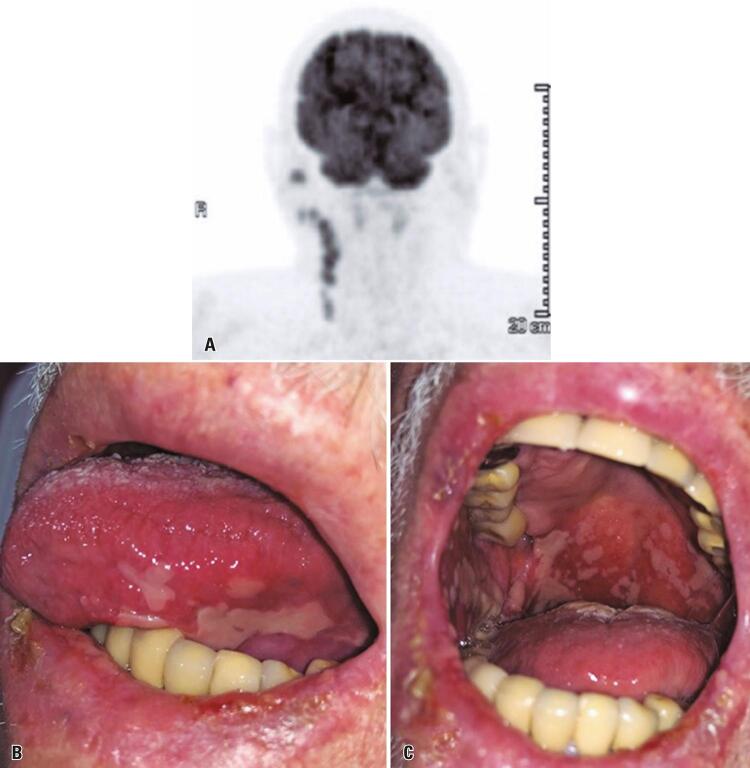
(A) baseline PET-CT showing FDG-avid right parotid and cervical lymph nodes. (B) and (C) clinical aspect of oral ulcers after two weeks of the first dose of cemiplimab. The lesions were extremely painful and the patient reported dysphagia
